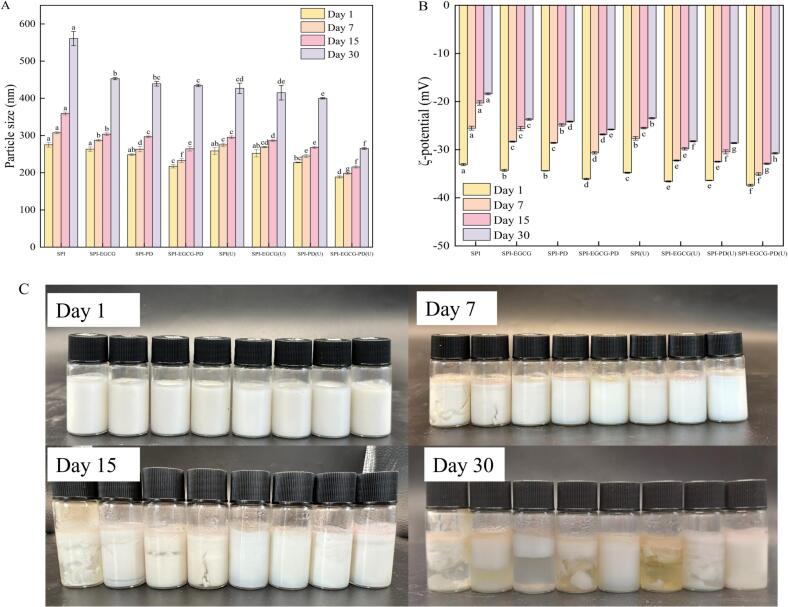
Particle size(A), ζ- potential(B), appearance(C) changes during storage of emulsions prepared using SPI, SPI-EGCG, SPI-PD, SPI-EGCG-PD, and their ultrasonic-treated samples.
